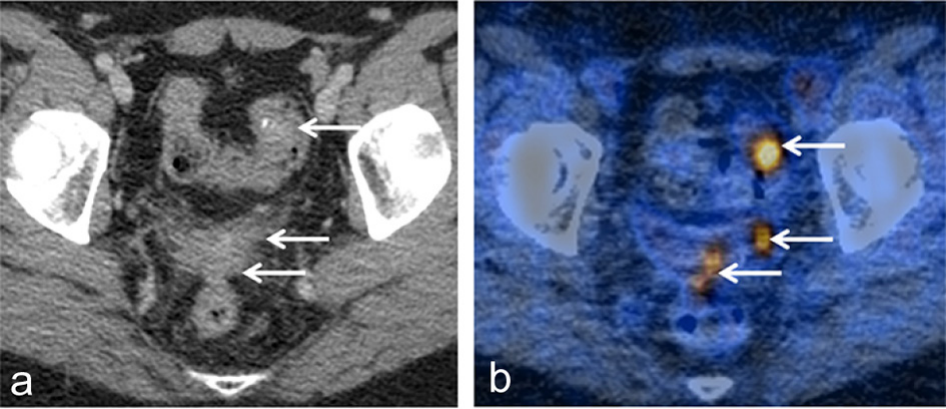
A 78-year-old female with previous serous papillary ovarian carcinoma, 2 years following radical surgery and R0 resection, presents with rising CA 125. (a) Part of diagnostic CT where no unequivocal site of recurrence was seen, but can be identified retrospectively (arrow). (b) Part of the^18^F-FDG PET CT demonstrating laparoscopically confirmed FDG avid disease along the serosal surface of the rectum, sigmoid colon and in the left hysterectomy bed (arrows). FDG, fluoro-2-deoxy-D-glucose; ^18^F-FDG PET CT, [fluorine-18]-fluoro-2-deoxy-D-glucose positron emission tomography. F-FDG PET CT, [fluorine-18]-fluoro-2-deoxy-D-glucose positron emission tomography.
